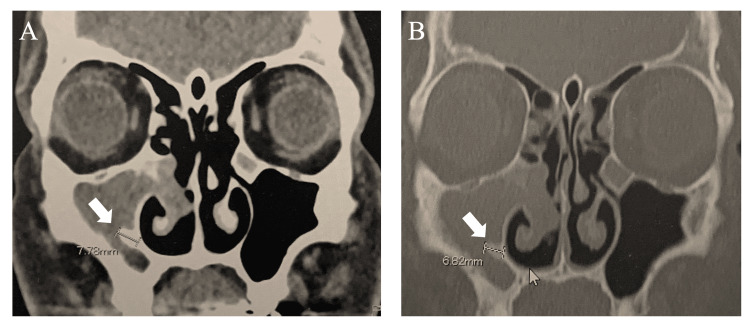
Bone hyperostosis at the IP attachment site on CT (A) Soft tissue density settings. (B) Bone window settings. Bone hyperostosis is observed in the medial wall of the maxillary sinus (indicated by the white arrow). CT: computed tomography, IP: inverted papilloma
